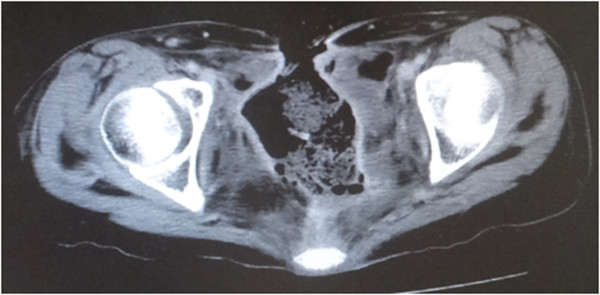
Computed tomography scan. The pelvic cavity, which extended from the pubic symphysis to the coccyx internally and communicated, is shown.
